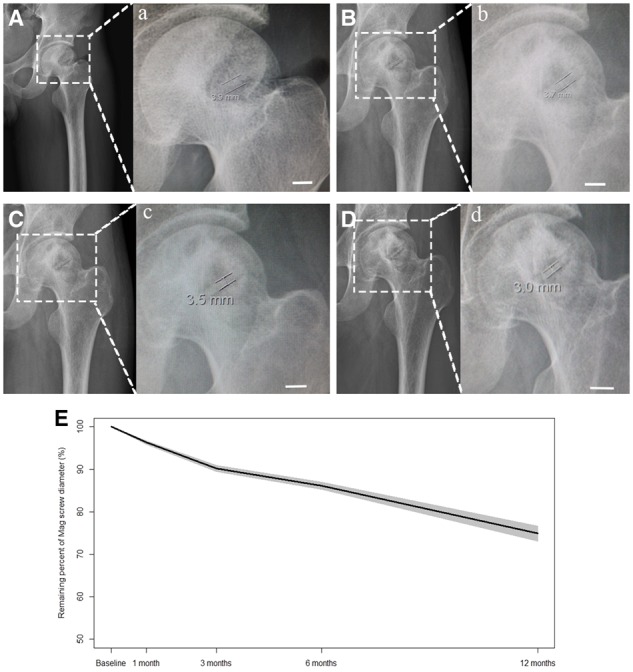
The temporal changes in biodegradation rate of Mg screws interpreted in decrease in screw diameter. (A–D) X-ray imaging of femoral head in patients performed with Mg screws at 1 (A), 3 (B), 6 (C), and 12 (D) months post-operatively. (a–d) Magnified surgical regions in (A–D) for measurement of screw diameter at different time points. Scale bar represents 10 mm. (E) The remaining percentage in screw diameter over implantation time. The black line stands for means while the grey area represents 95% confidence interval. The average reduction percentage of Mg screw diameter was 3.7%, 9.3%, 13.7%, and 25.2% at 1, 3, 6, and 12 months, posto-peratively. Reprinted from Ref. [73] with permission Figure 6.
